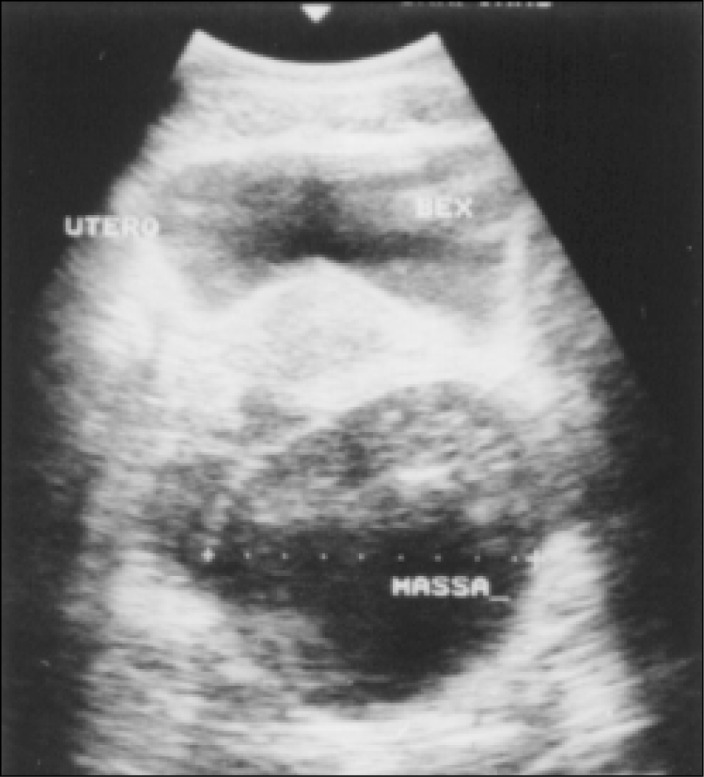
Case 1, adnexal cystic mass seen on ultrasound.
